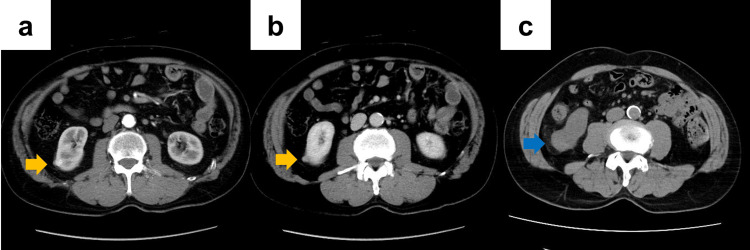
Computed tomography findings in Case 1 (a, b) Initial dynamic contrast-enhanced CT demonstrates a 10-mm exophytic tumor located at the lateral portion of the lower pole of the right kidney (a: early phase; b: portal venous phase). (c) After seven years of active surveillance, the tumor has increased in size to 30 mm. Arrows indicate the renal tumor.
